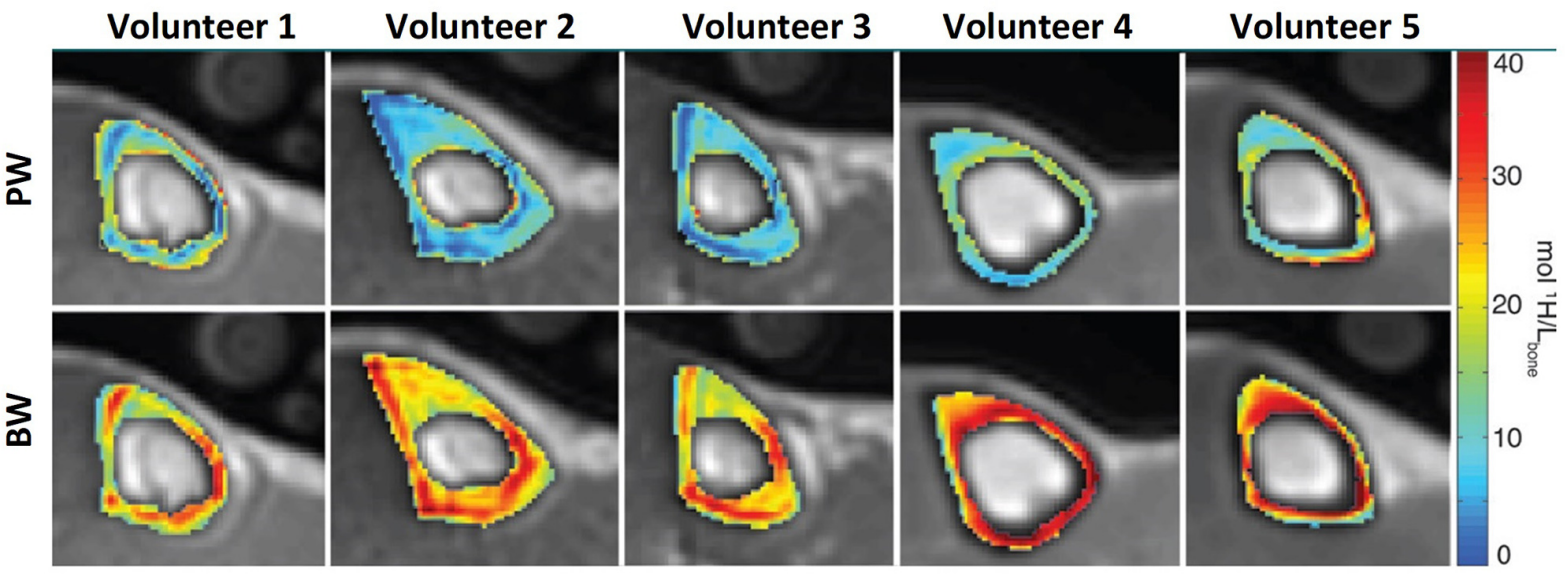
PW (top row) and BW (bottom row) proton density maps of tibial midshaft in five different subjects (two men and three women aged 24, 24, 49, 30, and 26 years). Maps are overlaid on UTE MRI images. This figure was previously presented by Manhard et al. (41). Reprinting permission is granted from Radiology journal (RSNA). This figure is modified for presentation purposes. Minor modifications were performed for presentation purposes.
